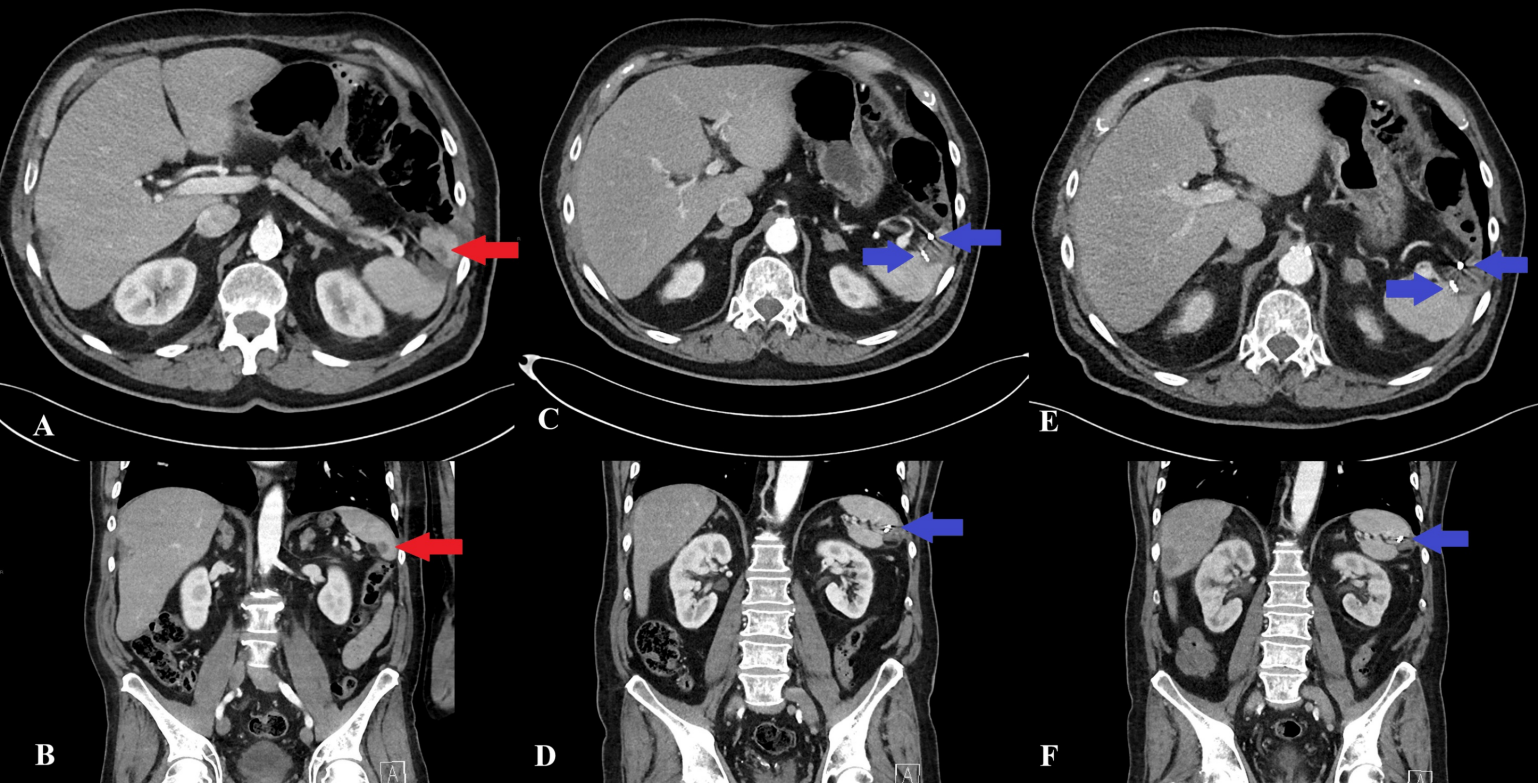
Computed tomography before and after fiducial placement Contrast-enhanced axial and coronal computed tomography scans of the abdomen pre-fiducial marker placement (A-B), post-fiducial marker placement (C-D), and the last follow-up control scan (E-F). The red arrows show the lesion, next to the ablation cavity. The blue arrows show the fiducial markers in the spleen.
